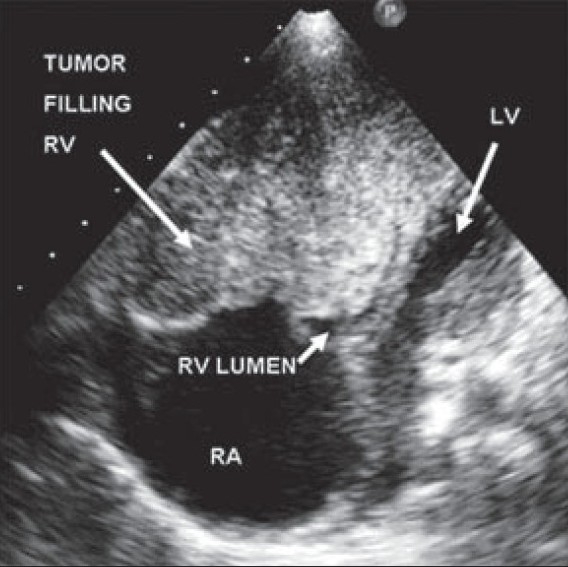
Echocardiogram in apical 4 – chamber view; RA, right atrium; RV, right ventricle; LV, left ventricle
